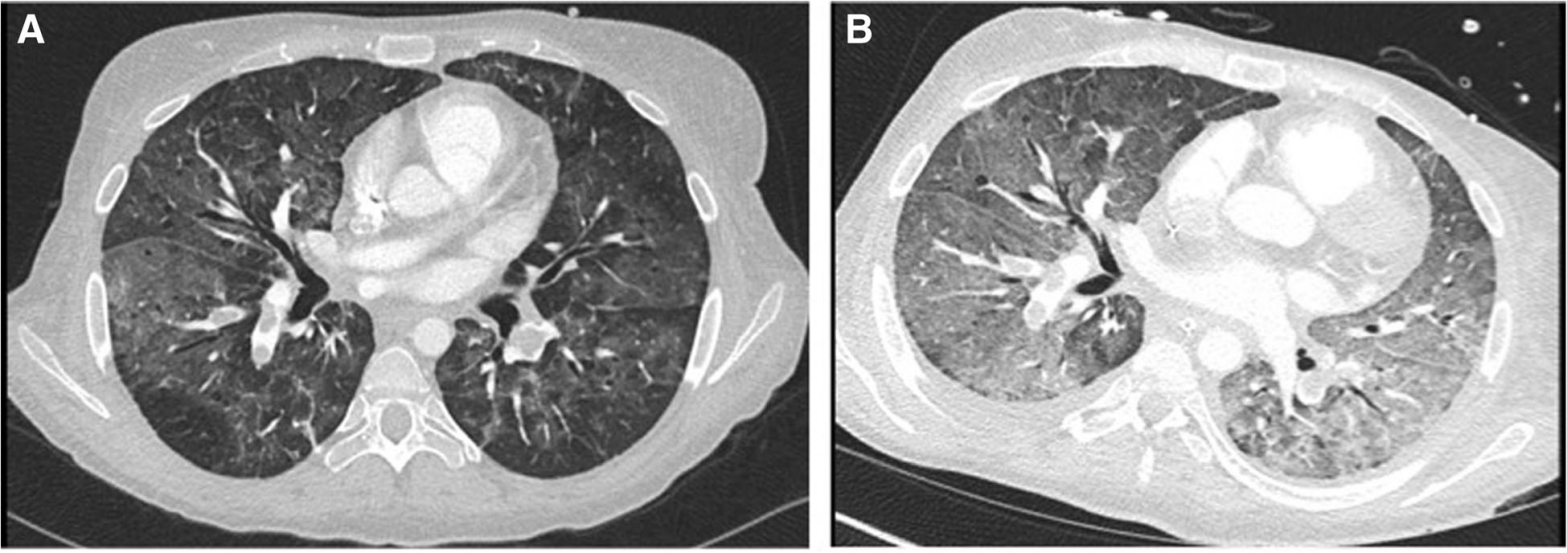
a Computed tomography scan of chest on admission showing bilateral central pulmonary embolism and diffuse ground glass opacities. b Computed tomography scan of chest after 3 days of non-invasive mechanical ventilation showing profound ground glass opacities
